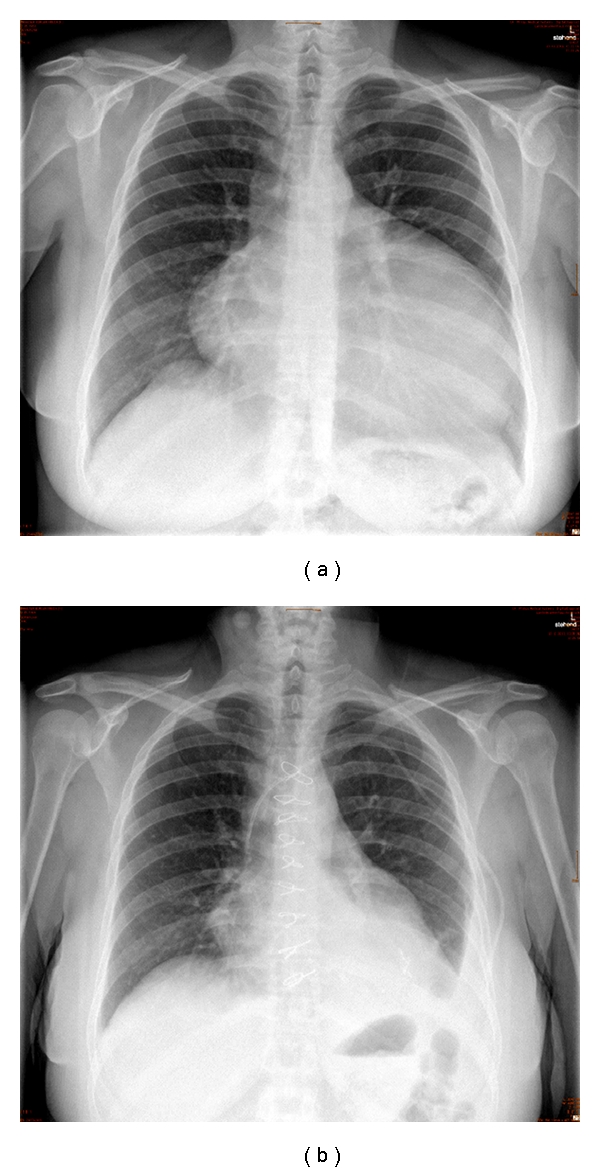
(a) Preoperative chest X-ray with enlargement of the cardiac silhouette. (b) Postoperative chest X-ray after removing the tumour mass.
